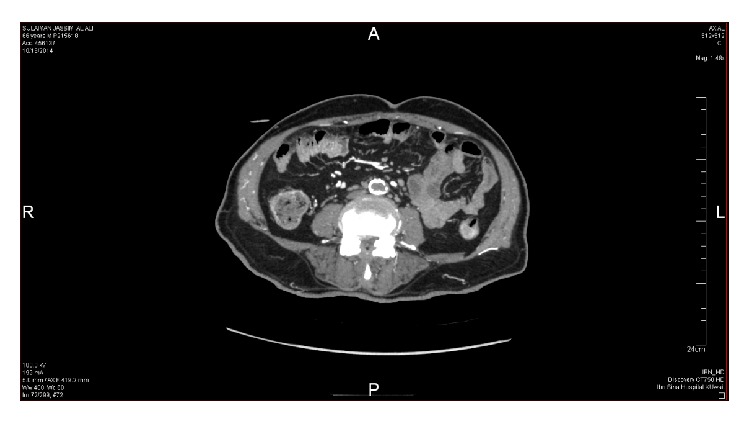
Thrombosis and calcification of the abdominal aorta on computed tomography with angiographic study (axial view).
